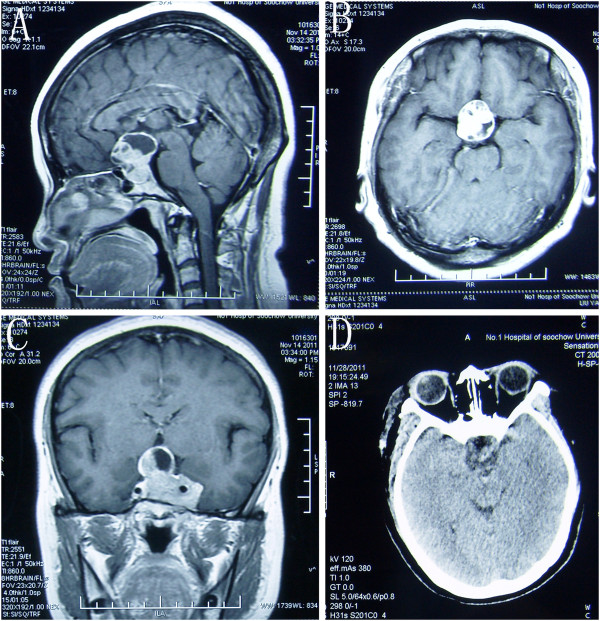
Case number 9: Sagittal (A), axial (B), and coronal (C) view of contrasted magnetic resonance imaging showing a pituitary adenoma. (D) Postoperative CT imaging of the same patient.
